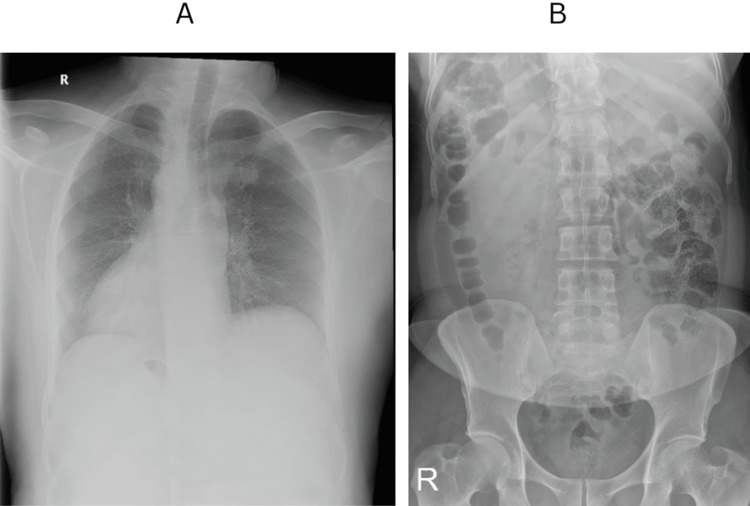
Posterior-anterior X-rays Posterior-anterior chest X-ray (A) and abdominal X-ray (B) showing dextrocardia and transposition of organs.
